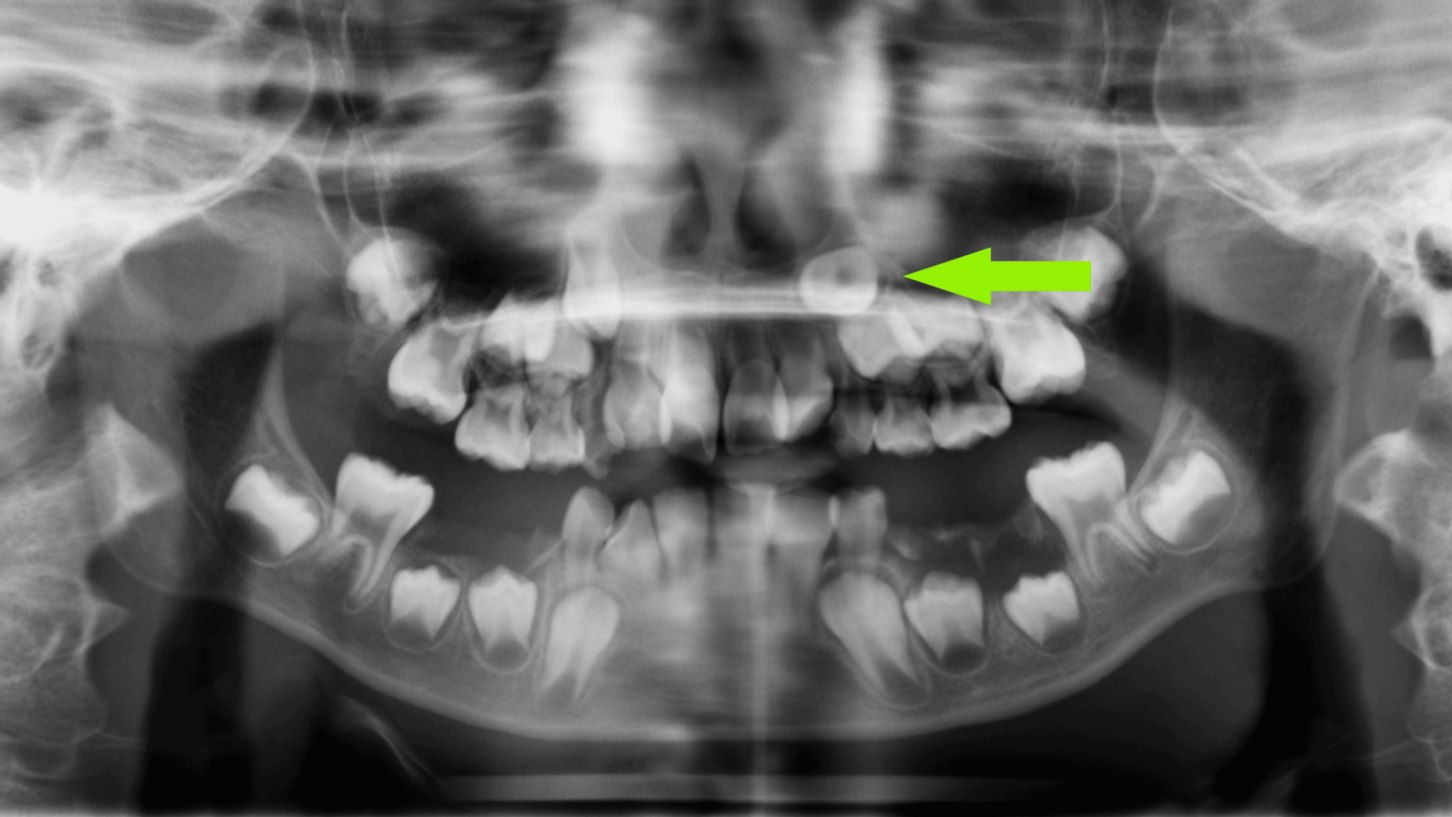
Panoramic radiograph taken five years before the start of treatment The tooth germ of tooth 23 was abnormally oriented and at risk of impaction (green arrow).
